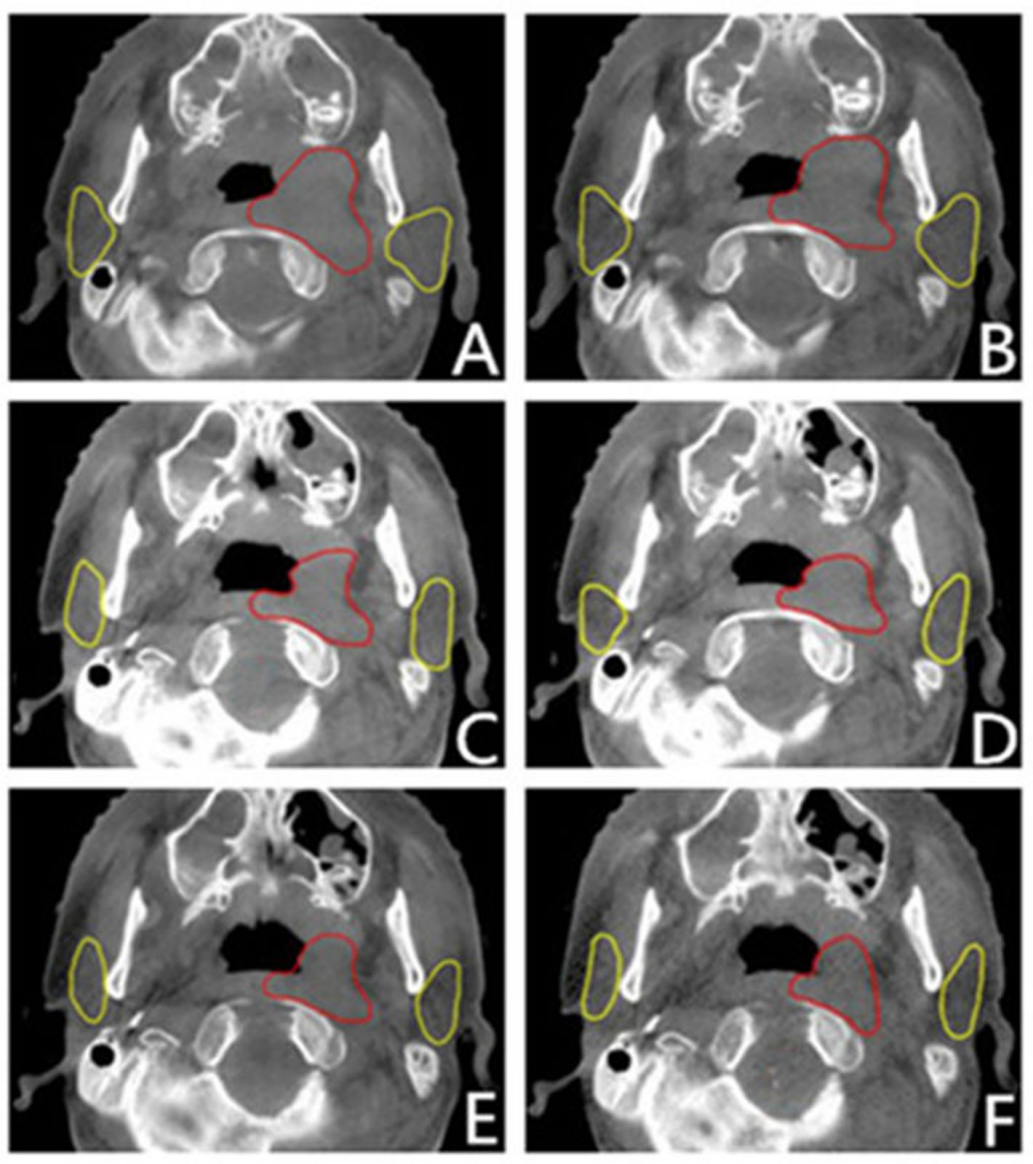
CBCT images performed before the 1 ^st^, 6^th^, 11^st^, 16^th^, 21^st^ and 26^th^ radiation time from one patient, corresponding to letter A, B, C, D, E, F. (In this level, the GTV and parotid glands were showed typically and clearly. The red outlines were GTV, and the yellow were parotids.)
